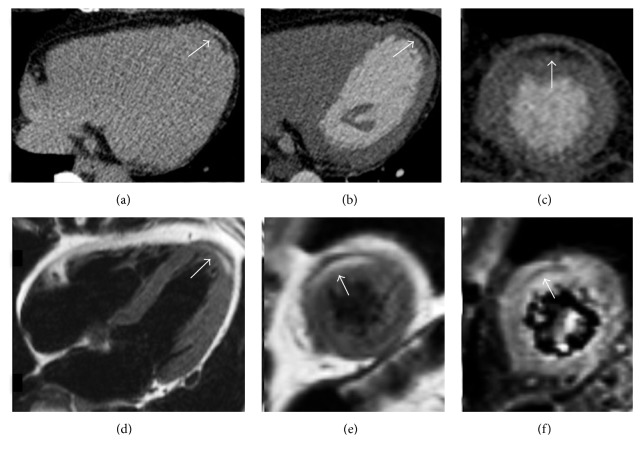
Thoracic noncontrast CT axial image in a 45-year-old female without any cardiac disease incidentally reveals a curvilinear hypodense stripe located within the subepicardial layer of the myocardium at the left ventricular apex, with negative attenuation values (a). The finding is successively confirmed at a cardiac CT postcontrast medium administration in axial view ((b) arrow) and in short axis multiplanar reconstruction ((c) arrow). In (d–f), the corresponding CMR exam, requested for a further evaluation, confirms the presence of the hyperintense stripe at the same place on T1-weighted black blood in four chambers ((d) arrow) and in short axis view ((e) arrow), with associated nulled signal on fat-suppressed T2-weighted image ((f) arrow), according to physiologic left ventricular apical fatty tissue.
